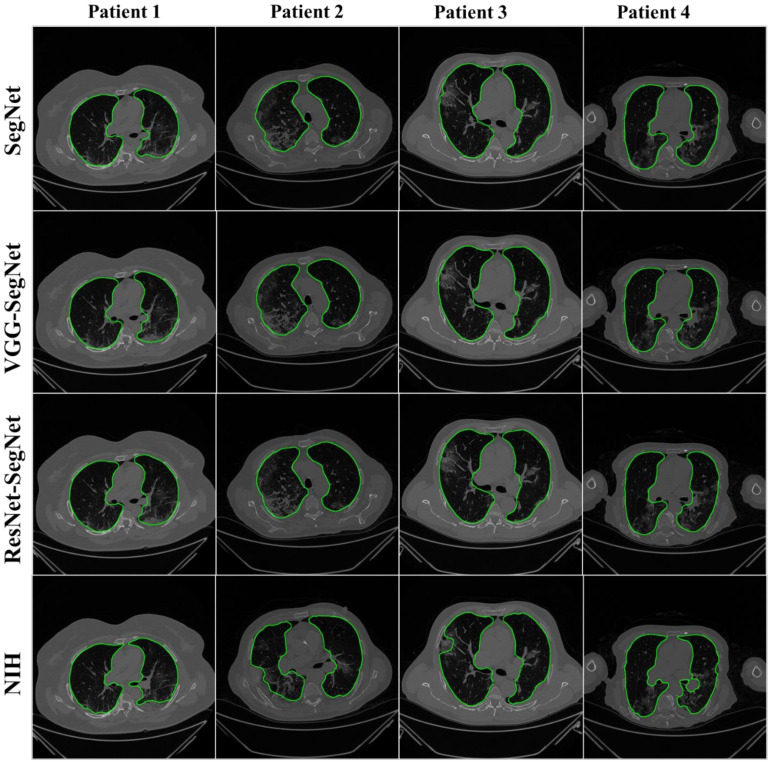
Results from the four AI models and NIH with a grayscale CT slice. Row 1 to Row 4 is four models: SegNet, VGG-SegNet, ResNet-SegNet, and NIH.
